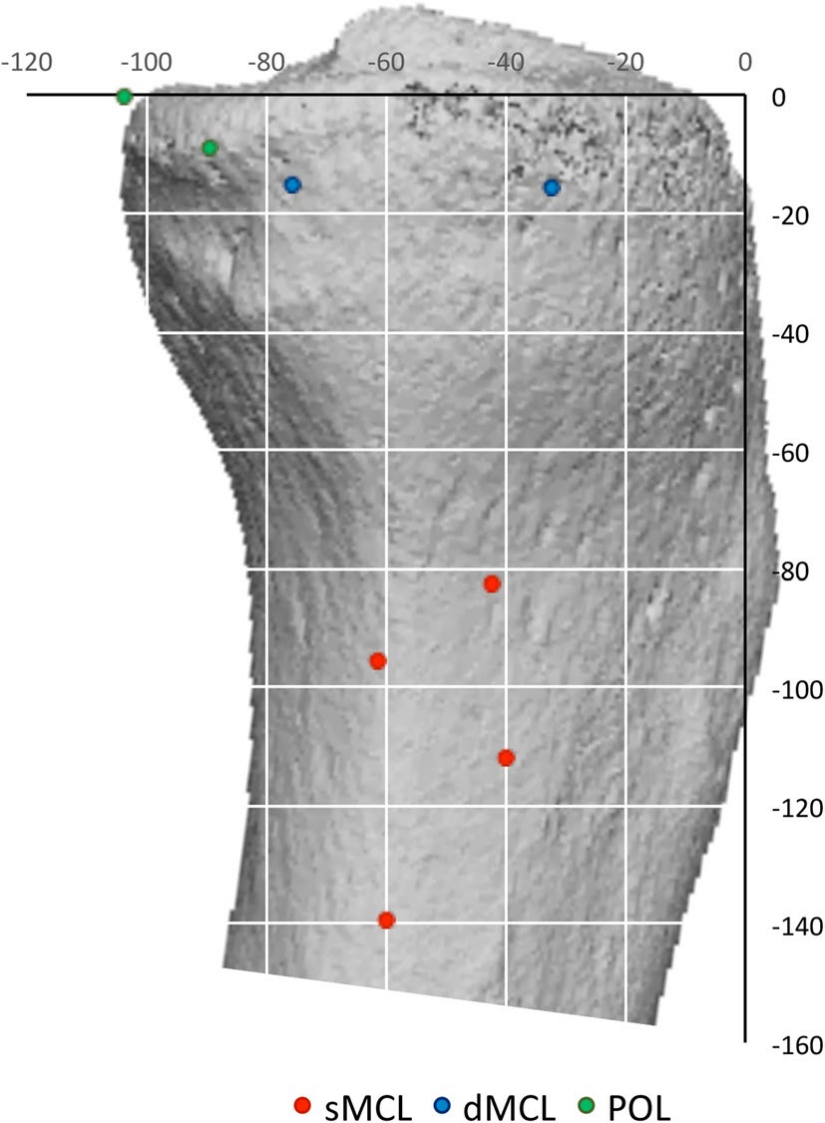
Mean tibial attachment points of the superficial medial collateral ligament (sMCL), deep medial collateral ligament (dMCL) and posterior oblique ligament (POL) from optical digitisation measurements. Data are superimposed onto a representative CT reconstruction. Data from the radiographic analysis (Table 2) were effectively the same when drawn in this manner, so are not shown
